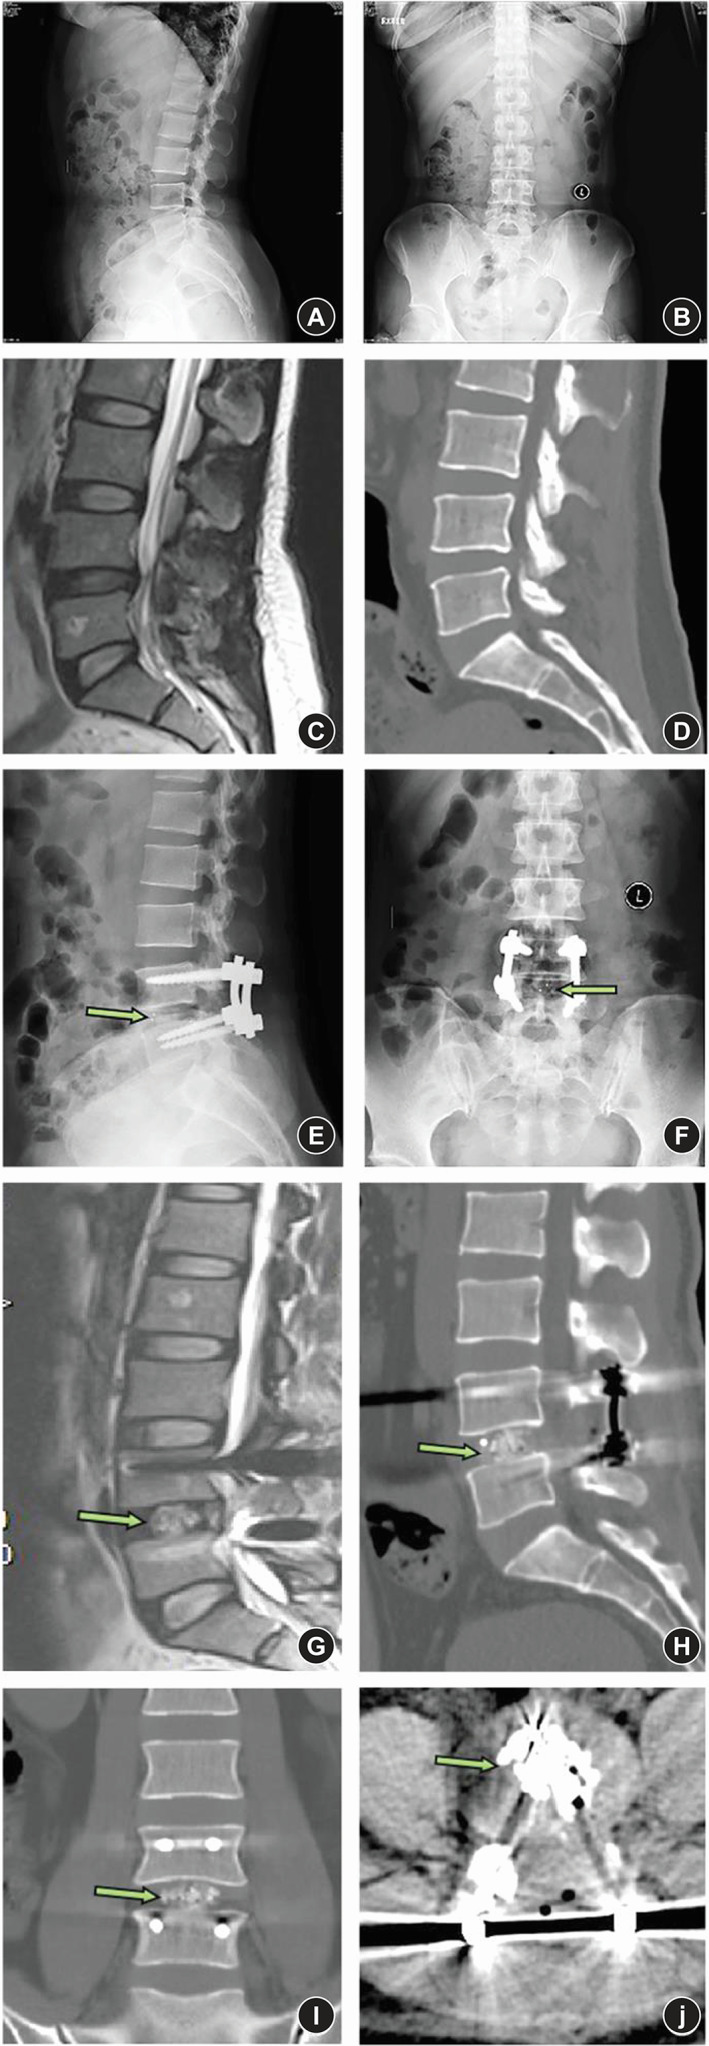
Case 1 (MI‐TLIF). (A) Sagittal X ray image before MI‐TLIF. (B) Anteroposterior X ray image before MI‐TLIF. (C) Sagittal MR image before MI‐TLIF. (D) Sagittal CT image before MI‐TLIF. (E) Sagittal X ray image after MI‐TLIF. The green arrow shows the marker of the cage. (F) Anteroposterior X ray image after MI‐TLIF. The green arrow shows the marker of the cage. (G) Sagittal MR image after MI‐TLIF. The green arrow shows the cage. (H) Sagittal CT image after MI‐TLIF. The green arrow shows the cage. (I) Coronal position CT image after MI‐TLIF. The green arrow shows the cage. (J) Cross section CT image after MI‐TLIF. The green arrow shows the cage
